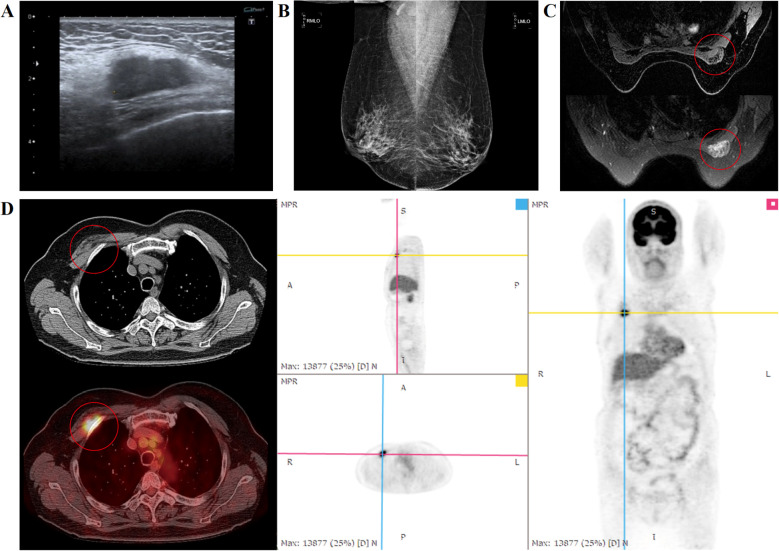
Occult Breast Cancer accompanied by interpectoral (Rotter’s) lymph node. (A) Ultrasound showing an enlarged interpectoral lymph node. (B) Mammography showing an enlarged interpectoral lymph node. (C) MRI showing enlarged interpectoral lymph node. (D) PET/CT showing an enlarged interpectoral lymph node, radioactively concentrated (circle), as well as no evident metabolically active lesion in the bilateral breasts.
